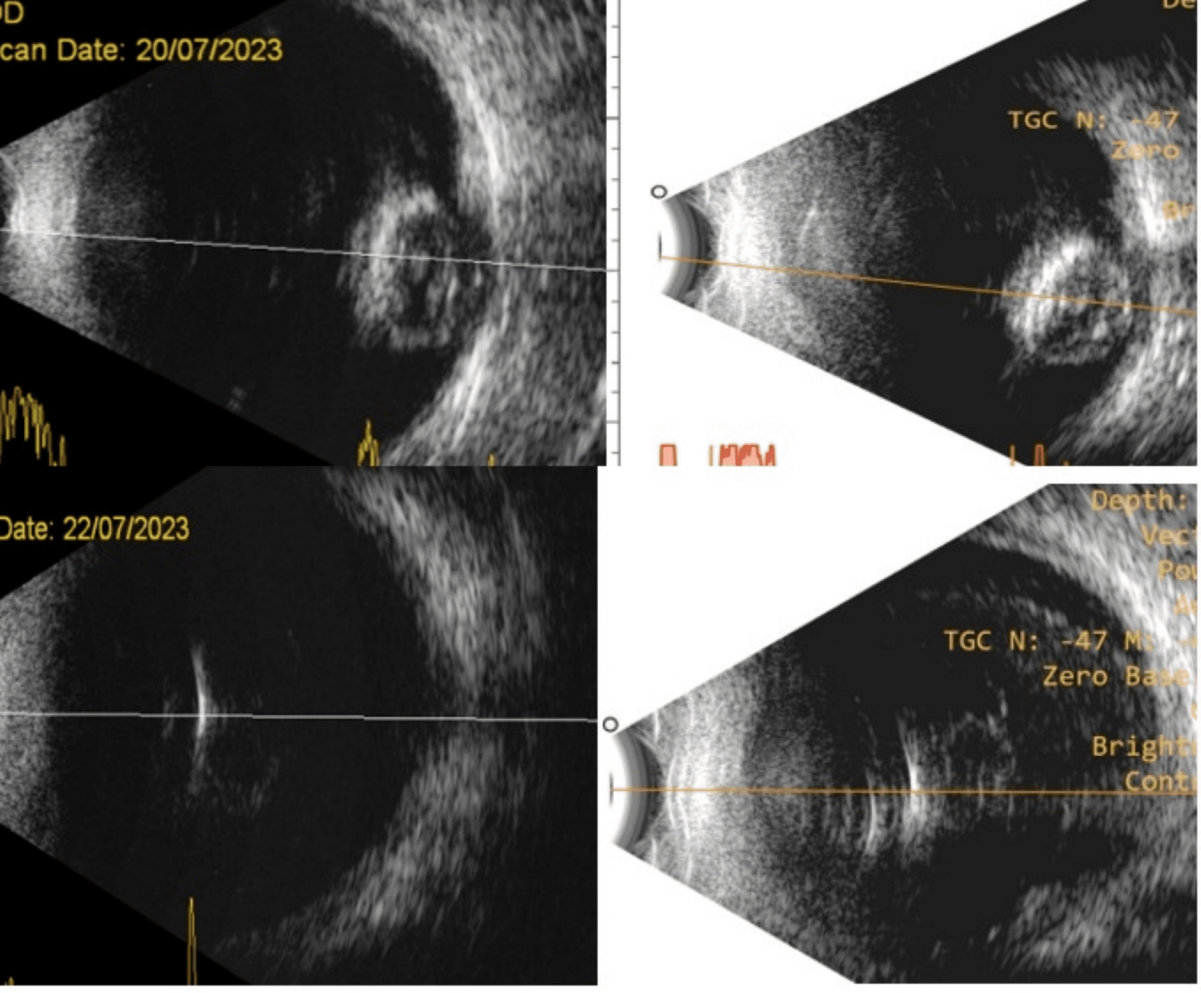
B-scan ultrasound in two ultrasonograms: (left) multinational-made device image and (right) India-made device image Top: Nucleus drop. Bottom: Intraocular foreign body
